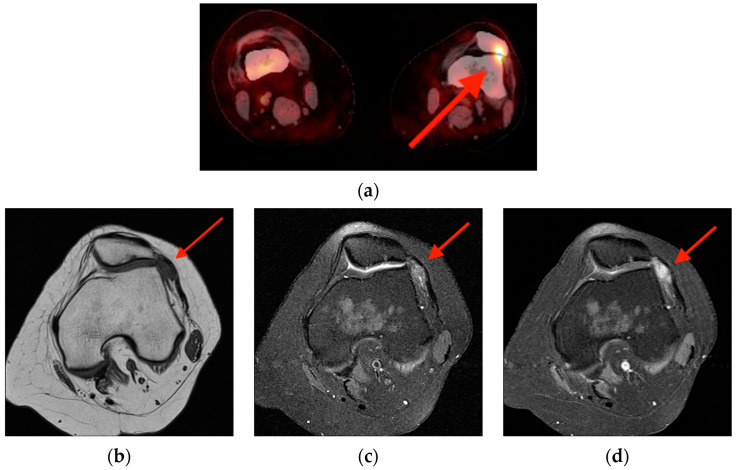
(a) FDG PET/CT obtained in a 43-year-old woman with head and neck cancer shows focal uptake within the lateral aspect of the left knee joint in the patellofemoral compartment (red arrow). This may be easily attributed to motion artifact or osteoarthritis; however, note the intra-articular location. MRI obtained eight months later shows an intra-articular soft tissue lesion with diffuse low signal intensity on T1-weighted images (b), heterogenous but primarily low signal intensity on T2-fat saturation imaged (c), and diffuse enhancement on post-contrast images (d), characteristic of TGCT/PVNS (red arrows). FDG PET/CT = fluorine-18 fluorodeoxyglucose positron emission tomography/computed tomography. MRI = magnetic resonance imaging. TCGT/PVNS = tenosynovial giant cell tumor/pigmented villonodular synovitis.
